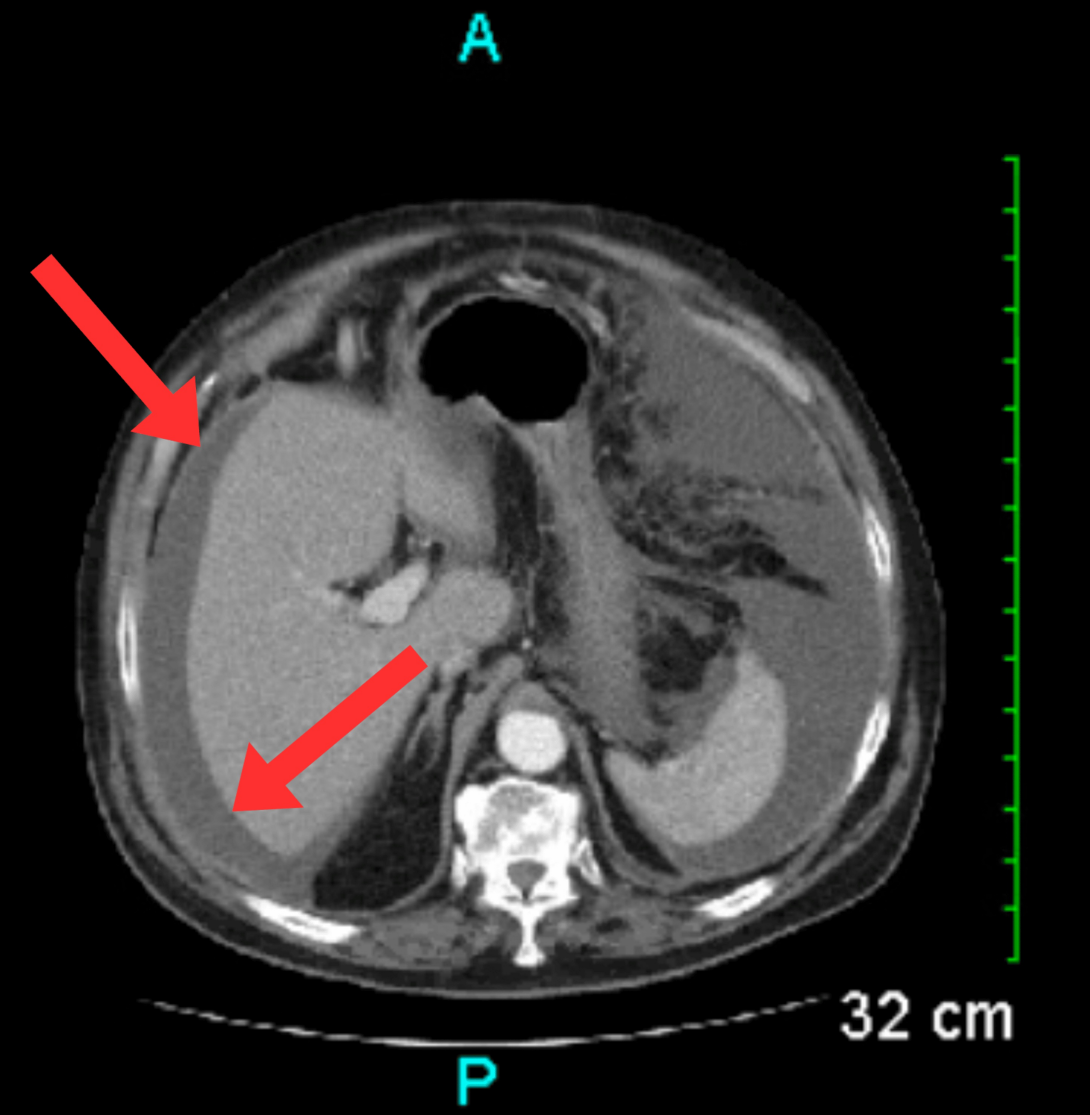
CT scan through the upper abdomen showing ascites (red arrows) adjacent to the liver. CT: Computed tomography
